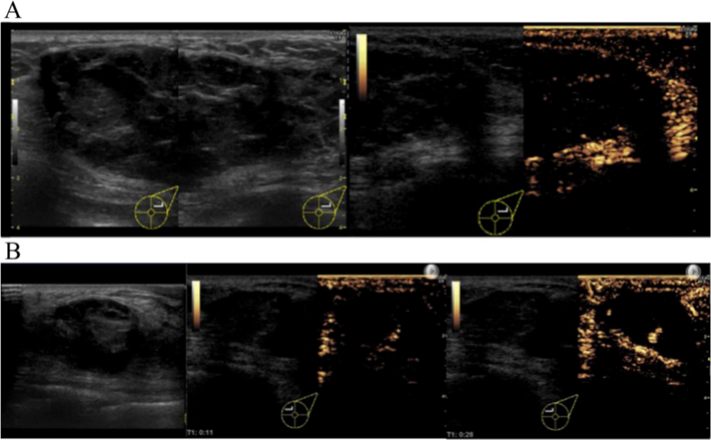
Ultrasound (US) and contrast-enhanced ultrasound (CEUS) were performed in case 1 (a, *upper line*) and case 2 (b, *lower line*) over 3 years after surgery. In case 1, the graft is detected as a circumscribed oval and heterogeneous low echoic mass on US. This mass is coated by a smooth capsule with a high echogenic structure (a, *left panel*). CEUS shows no blood flow into the mass from the outside fat tissue (a, *right panel*). In case 2, the graft is seen as a circumscribed oval and heterogeneous low echoic mass on US, very similar to case 1. CEUS shows blood flow into the graft mainly from the retro-mammary fat side, which begins approximately 10 s after intravenous administration of contrast agent (*center panel*). In the mass, several blood flows are observed with persistent enhancement without attenuation once the vessels are enhanced for 30 s after administration (*right panel*)
